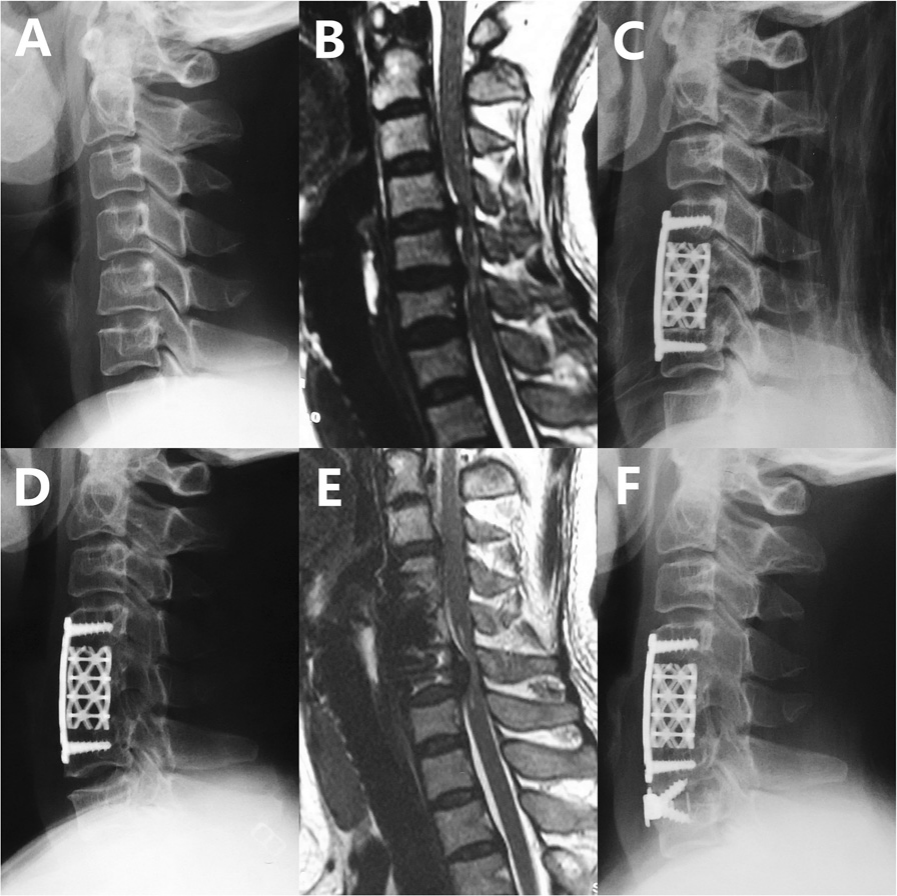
C4 to C7 DCS with recurrent myeloradiculopathy 6 years after C4-C6 anterior cervical decompression and fusion (ACDF). a Preoperative radiograph shows DCS at C4-C7 levels. b Preoperative MRI of this 49-year-old female shows severe compression of the spinal cord at C4-C6 levels. c Radiograph after initial surgery shows ACDF at C4-C6. d Radiograph 6 years after initial surgery shows osseous fusion of C4 and C6 and development of adjacent-segment degeneration (ASD) at C6-7. e MRI at 6-year follow-up shows development of ASD and spinal cord compression at C6-7. f Radiograph after the second surgery shows ACDF at C6-C7. ACDF, anterior cervical decompression and fusion; ASD, adjacent-segment degeneration; DCS, developmental canal stenosis, MRI, magnetic resonance imaging
